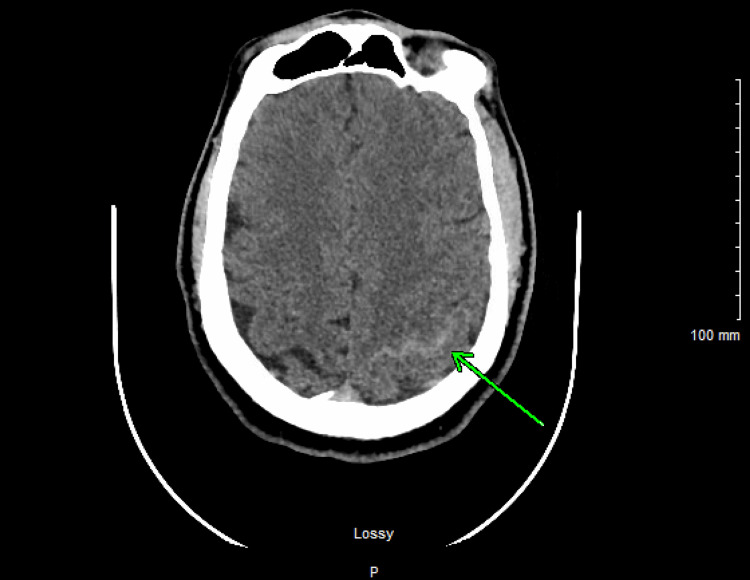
CT of the head demonstrating left-sided subarachnoid hemorrhage (green arrow)
